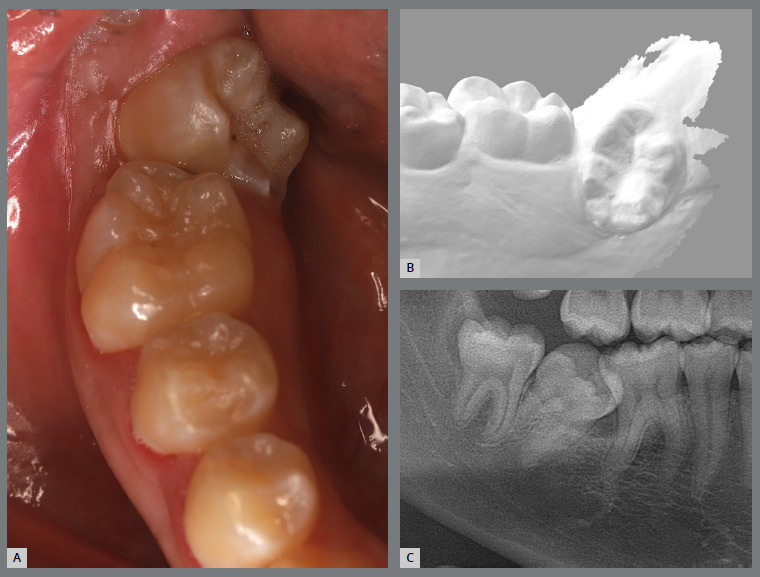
Images of impaction of the mandibular right second molar: A)close-up intraoral photograph; B) lingual view of the digital scan; and C)panoramic radiograph close-up.
